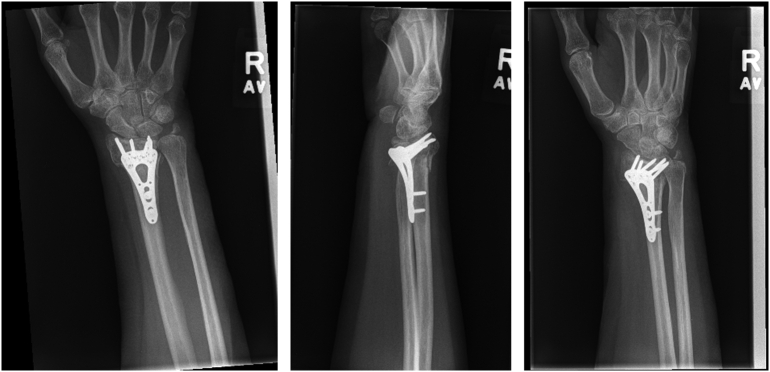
Postoperative radiographs at one month, demonstrating intact distal radius hardware with no evidence of loosening.
